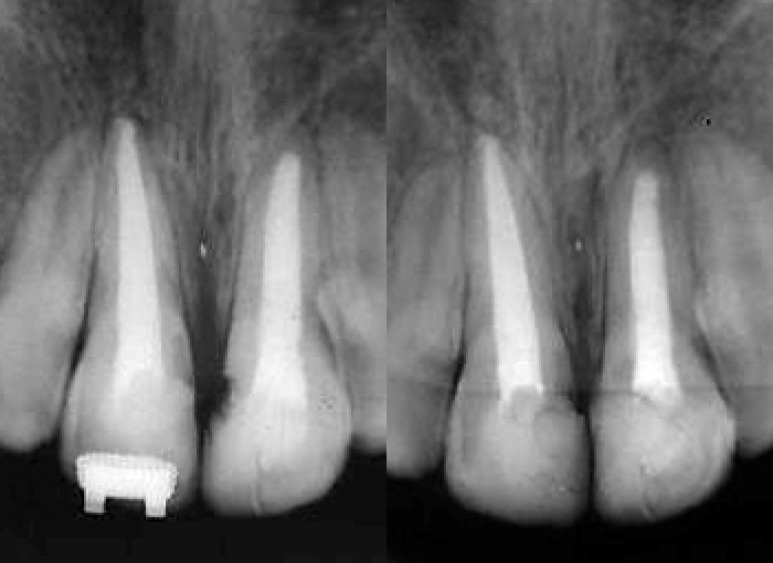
Radiographies at the end of the retention course (left) and 6-years follow up (right
